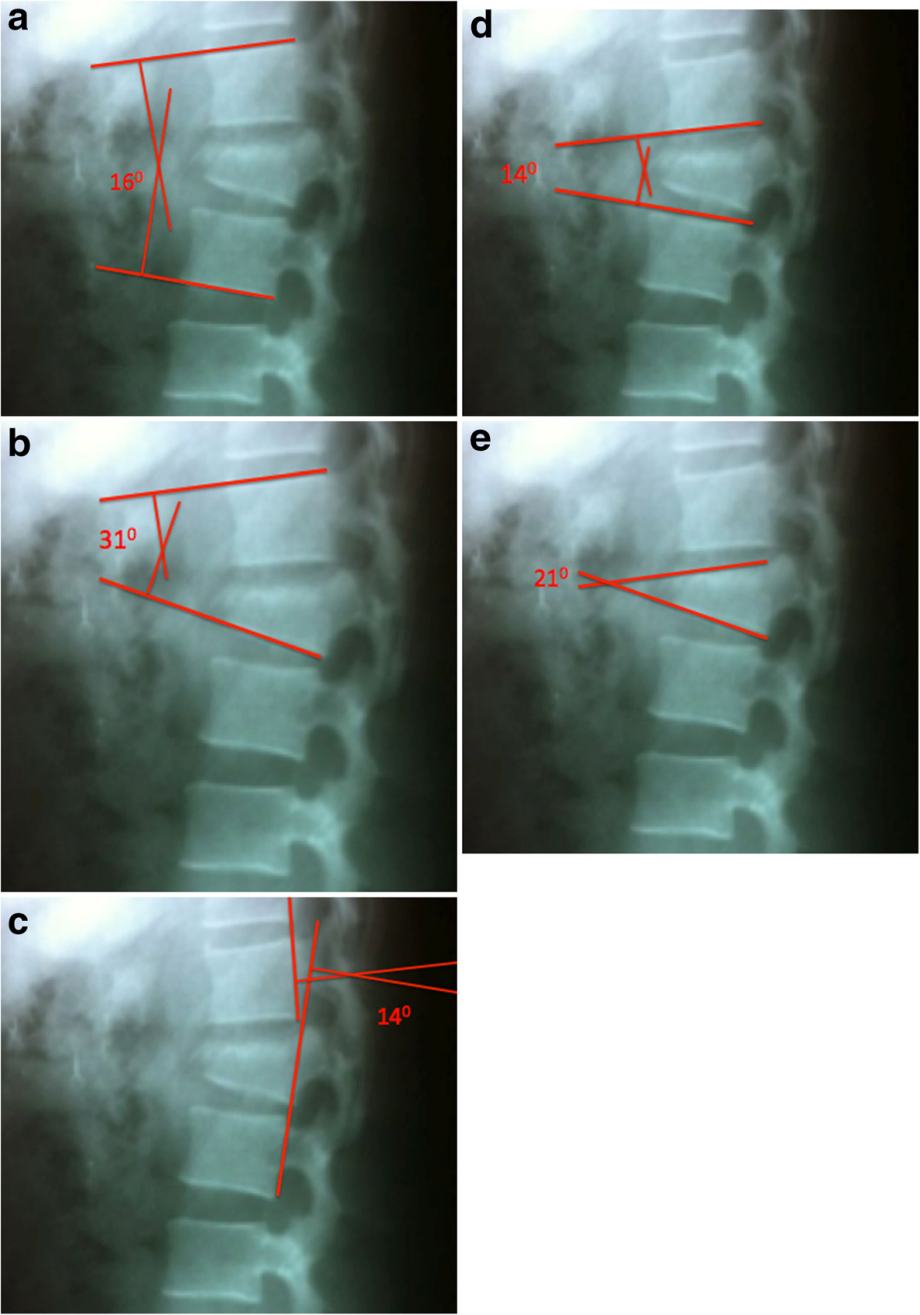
Radiography of the thoracolumbar transition with L1 fracture. Evaluation of segmental kyphosis by the five methods described: a) method 1, b) method 2, c) method 3, d) method 4 and e) method 5.
